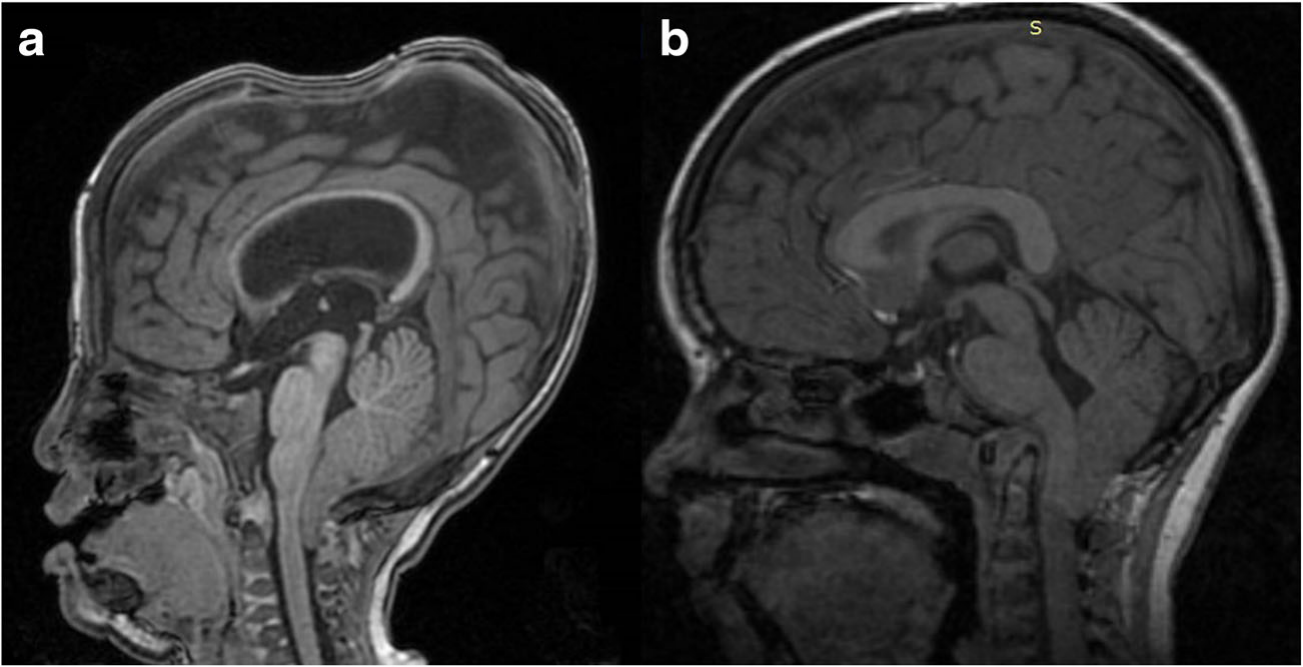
Sagittal MRI showing CIM as a result of two different conditions: a Crouzon syndrome (18-month-old girl), note the severe FCP hypoplasia, the marked verticalization of the tentorium, the upward and downward displacement of the cerebellum, and the sellar deformation of the neurocranium, b primary CIM associated with basilar invagination (5-year-old boy), the PCF hypoplasia is moderate but a significant kinging of the bulbo-pontine junction is present. In both cases, the foramen magnum is large enough to allow the hindbrain herniation
